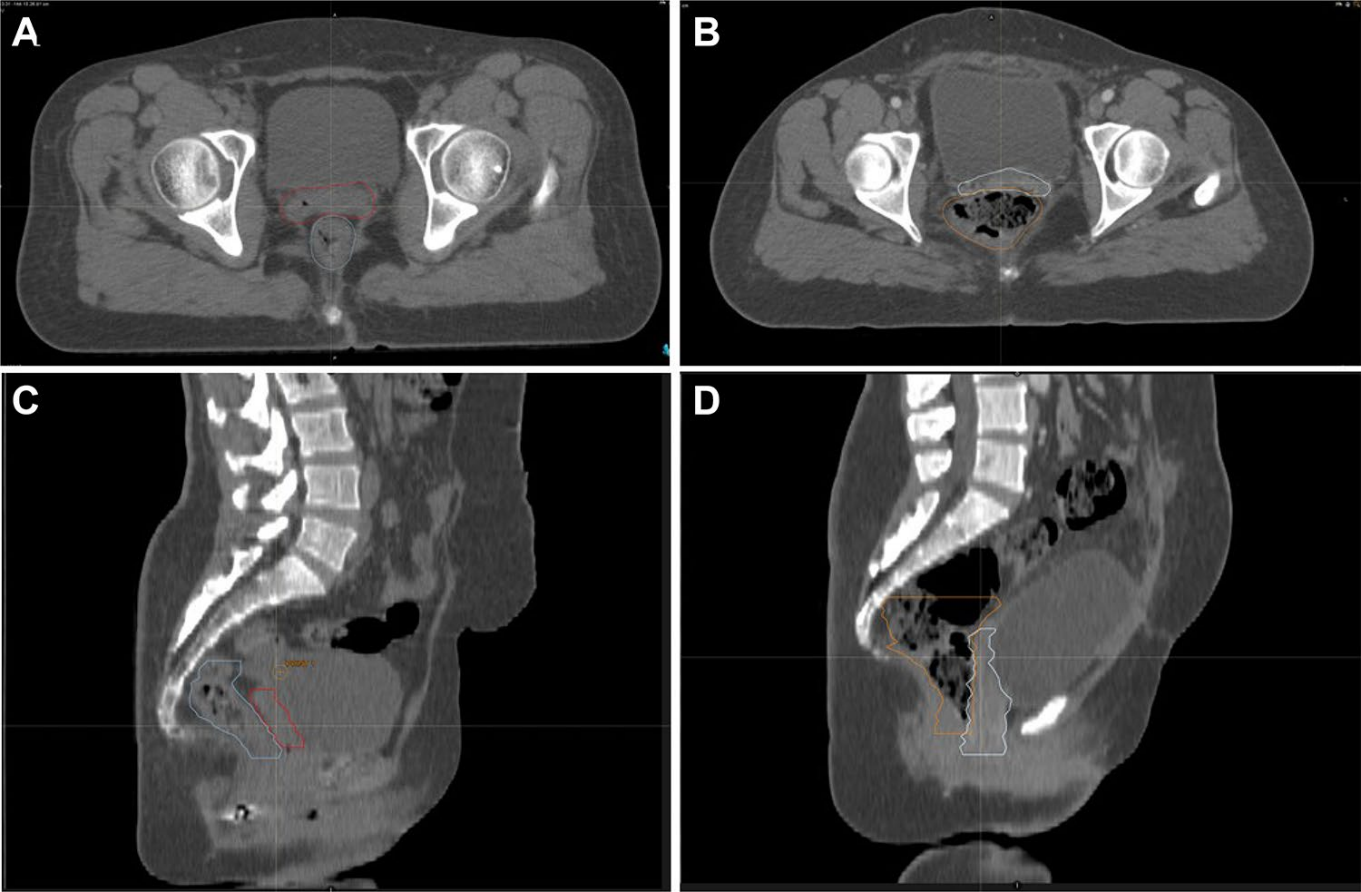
A representative example of cross-organ deformation is shown wherein a rectum filling has greatly impacted the cervix. The trans-axial and sagittal CT views of two patients with different rectum filling are shown. In A and C, the rectum with a normal filling has not affected the cervix. In B and D, the rectum filling has substantially deformed the cervix
